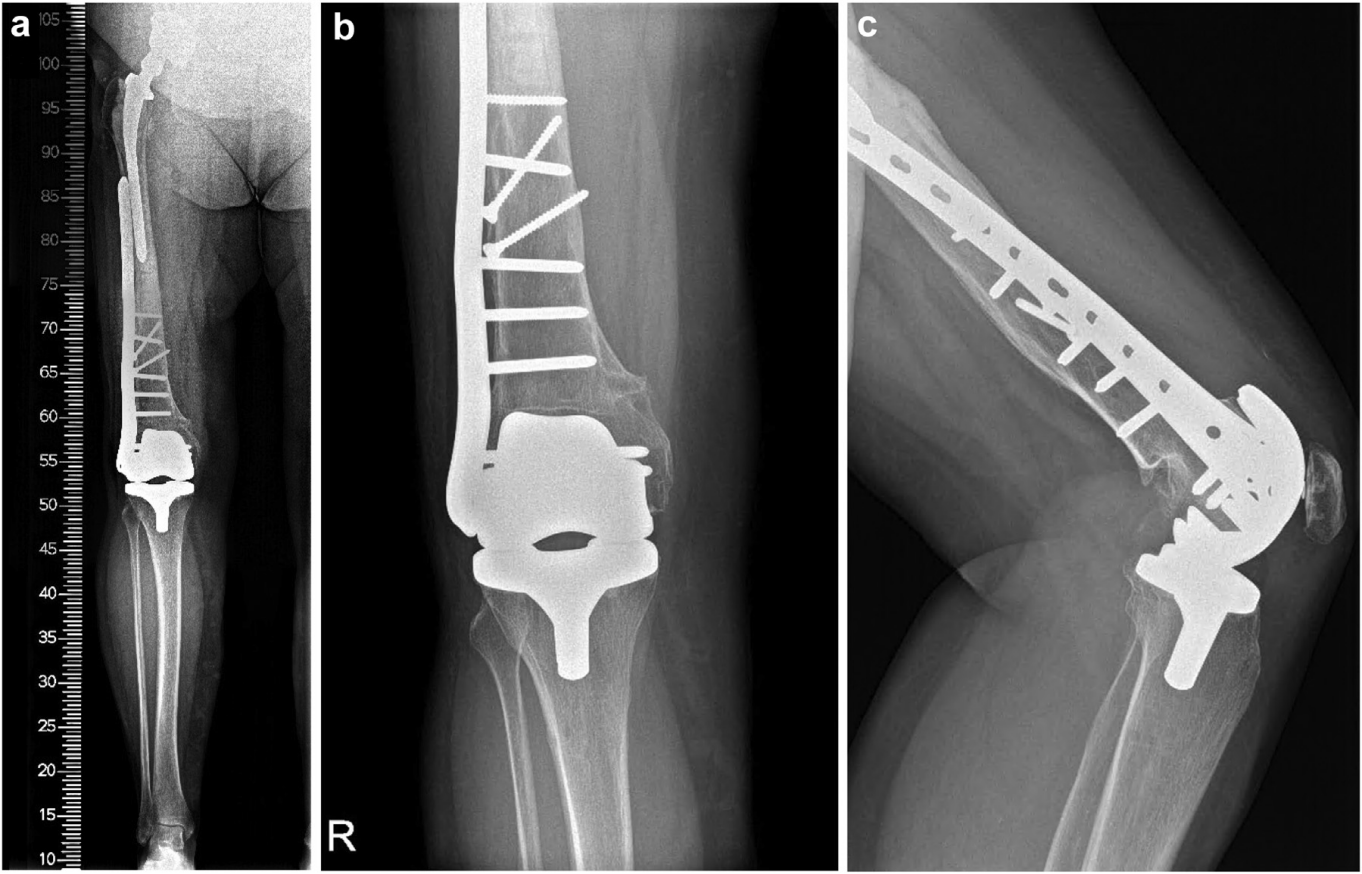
Six-week postoperative radiograph showing optimal alignment of the TKA and no signs of hardware loosening. (a) Large format, including the hip arthroplasty; (b) TKA detail, anteroposterior radiograph; (c) TKA detail, lateral radiograph.
